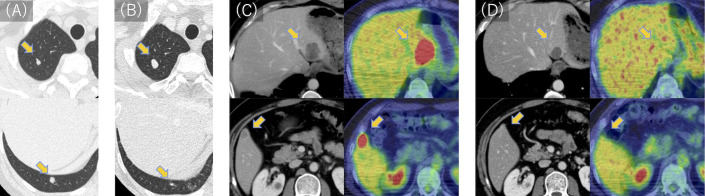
CT and PET scan images of lung and liver metastases during treatment. (A) Two lung metastases were found 3 months after wide resection. (B) Lung metastases were deemed stable following five courses of doxorubicin and ifosfamide. (C) Two liver metastases were identified in the left and right lobes 9 months after wide resection. (D) Following 19 courses of pembrolizumab, liver metastases were significantly reduced in the left lobe and became undetectable in the right lobe. Fluorodeoxyglucose uptake was reduced to physiological levels. PET, positron emission tomography; CT, computed tomography.
